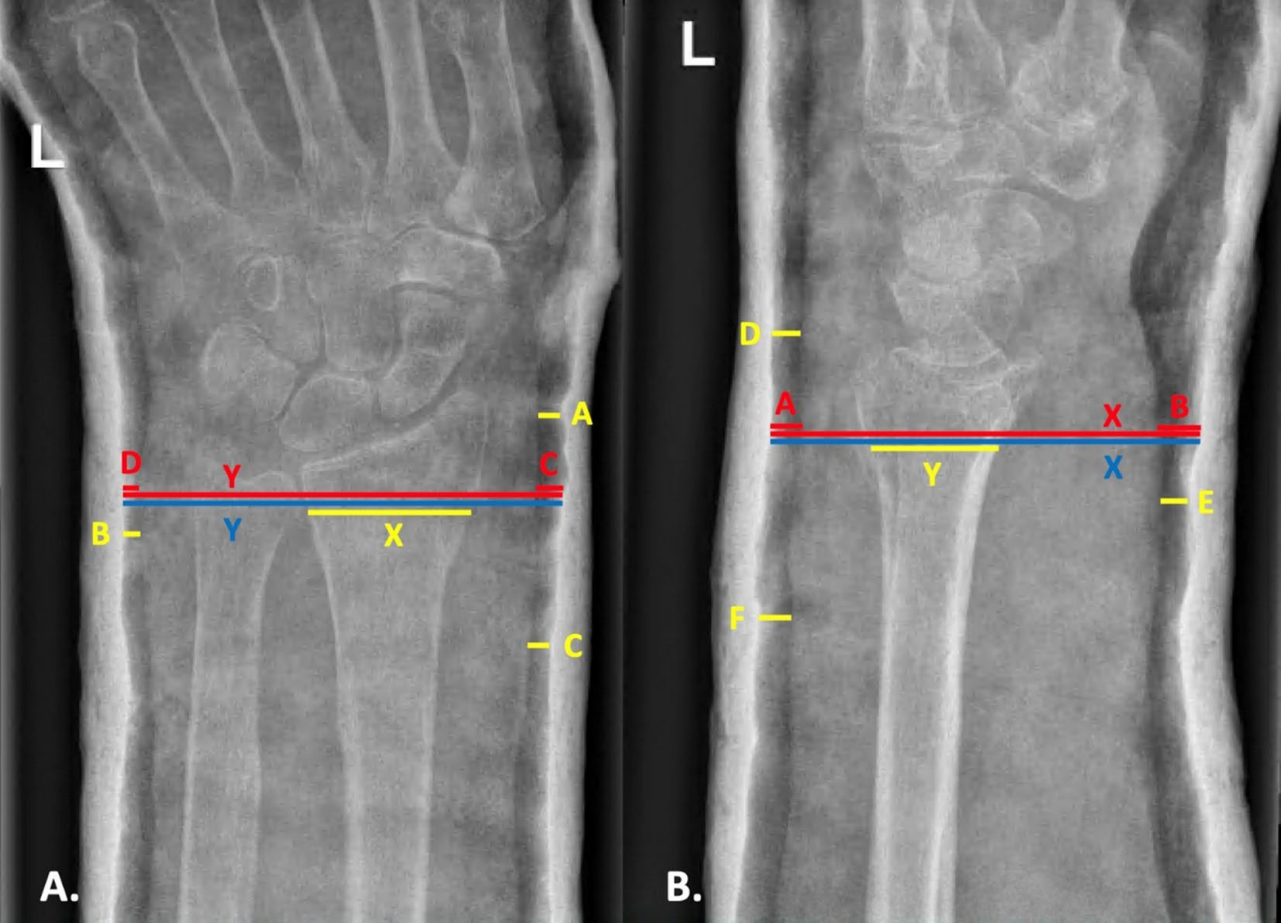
A. and B. Cast indices measurements shown on postero-anterior (A) and lateral (B) radiographs
